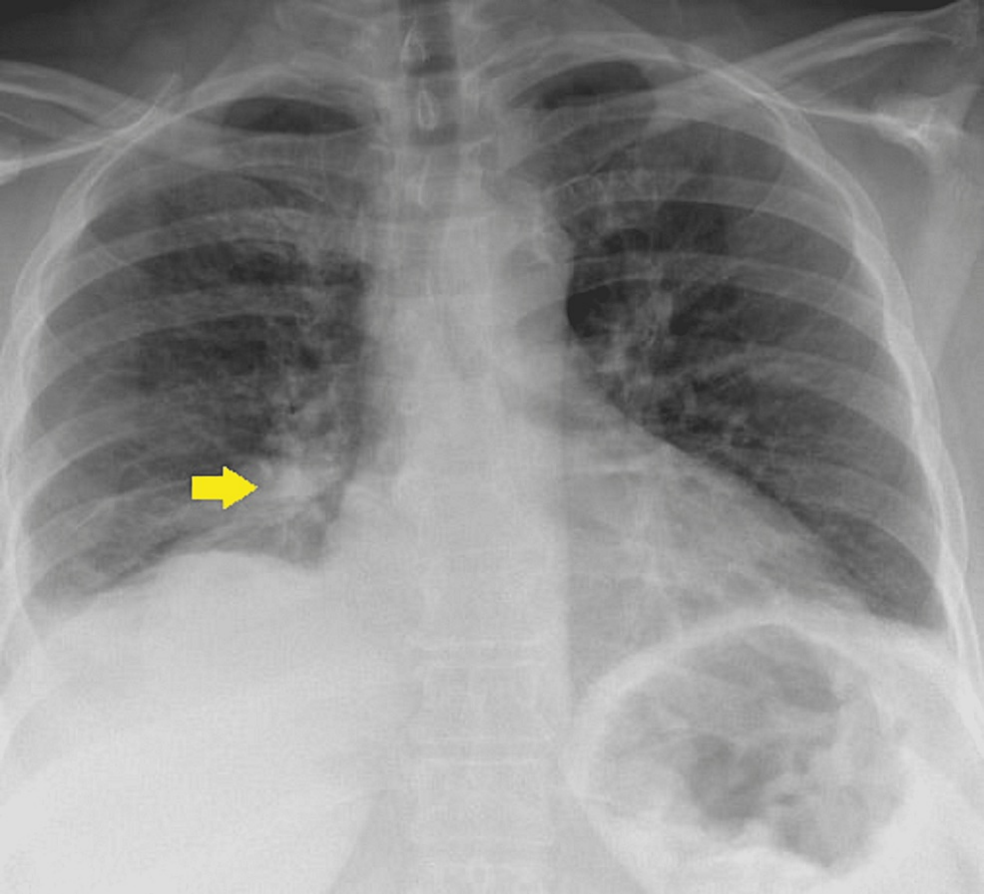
Chest x-ray showing right lung mass (arrow).
